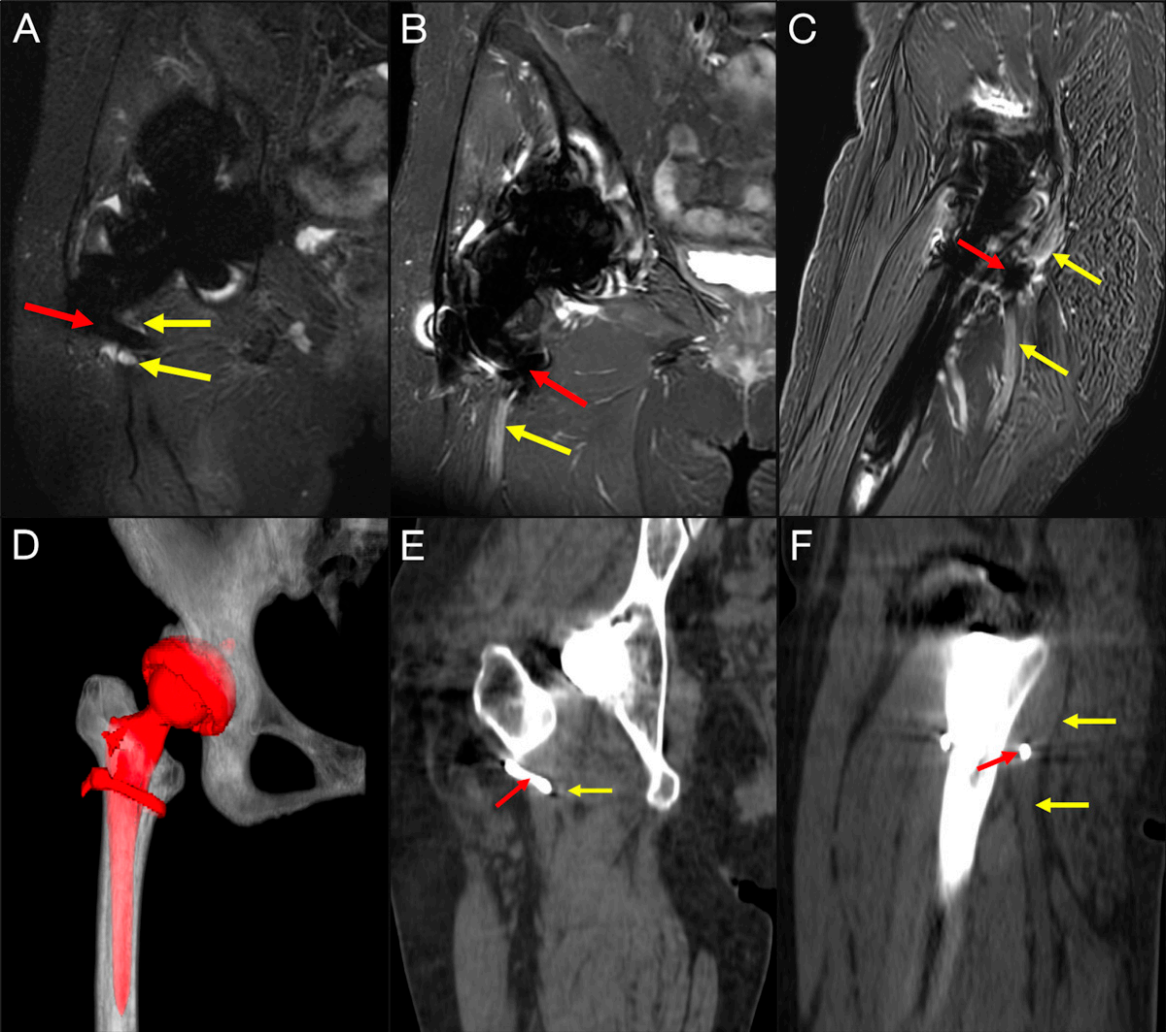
Preoperative magnetic resonance neurogram and computed tomography. Preoperative magnetic resonance neurogram showed abnormal features in the right sciatic nerve at the hip level. There is a discontinuity and abnormal thickening of the sciatic nerve (yellow arrow) with increased T2-weighted signal intensity adjacent and distal to the site of femoral cerclage wire placement (red arrow; metal artifact). (A and B) Coronal T2, left side of image = right side of patient; (C) sagittal T2, left side of image = anterior to patient. Preoperative computed tomography (CT) scan and three-dimensional reconstruction of the right lower extremity showed significant thickening of the sciatic nerve (yellow arrow) with the posterior aspect of the cerclage wire (red arrow) contiguous with the sciatic nerve. (D) Three-dimensional reconstruction of the right total hip arthroplasty implant and femoral cerclage wire in red color; (E) coronal CT, left side of image = right side of patient; and (F) sagittal CT, left side of image = anterior to patient.
